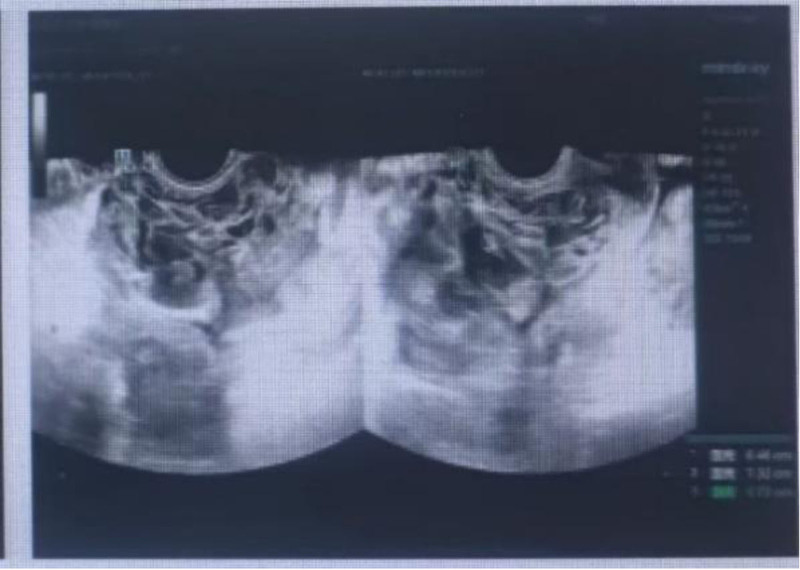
Ultrasound image showing a cluttered echo mass in the uterine cavity with fine, punctate cysts.
